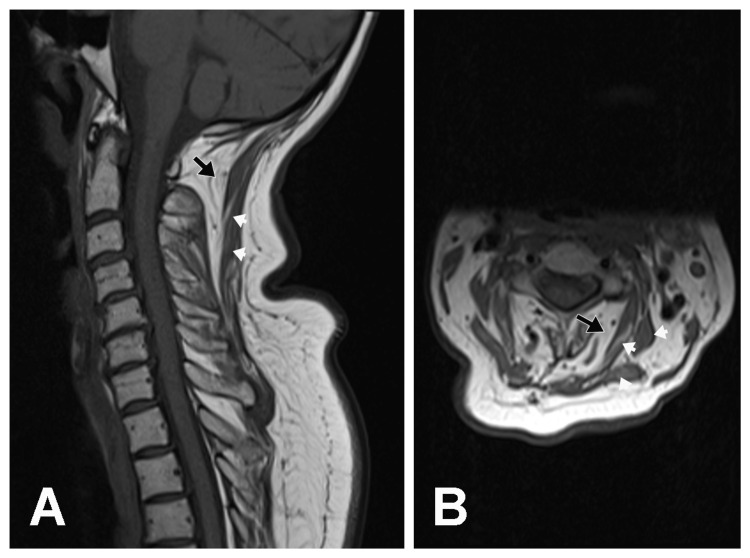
Sagittal and Axial T1-Weighted MRI Images of the Cervical Spine (A) Sagittal and (B) axial T1-weighted MRI images of the cervical spine demonstrating extensive fatty degeneration of paraspinal muscle (black arrows) and atrophic paracervical muscles containing white areas of fatty degeneration (white arrowheads).  Also note the radiation-induced fatty marrow replacement changes in the cervical spine.
